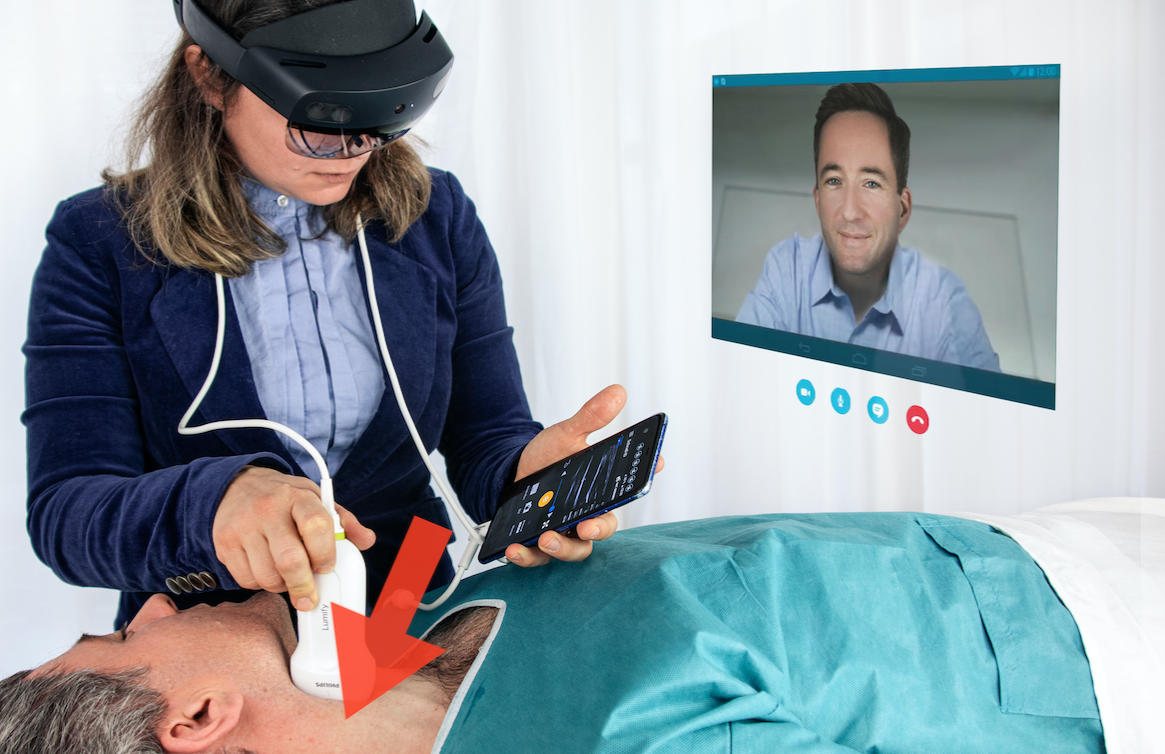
Example of the presented study setting with the investigator wearing the HoloLens 2 (Microsoft Corp) while performing the ultrasound examination with the Lumify transducer (Philips Healthcare).
